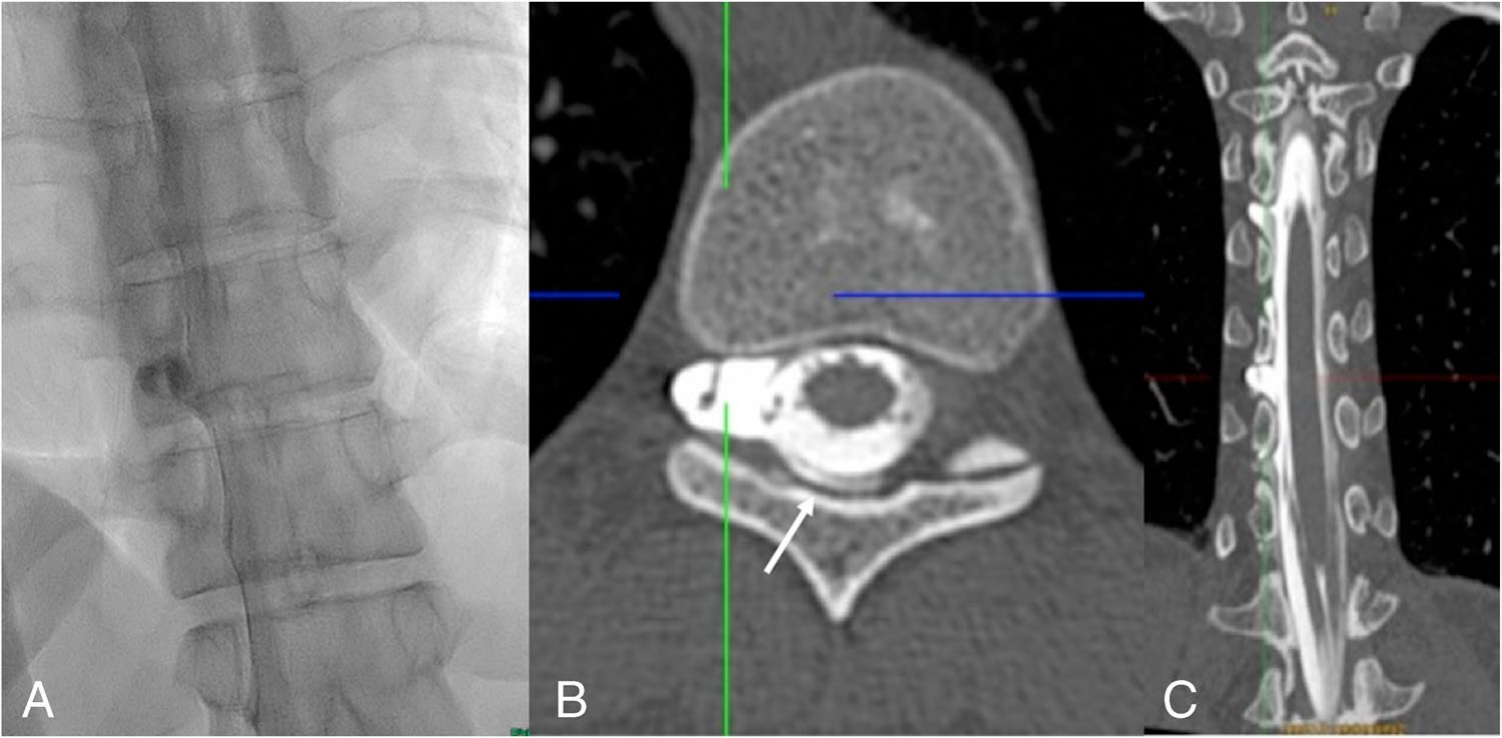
Type 2 leak: Right-sided lateral decubitus myelography shows a leaking meningeal diverticulum Th 10/11 on the right-side (A). Subsequent lateral decubitus CT myelography (B, C) shows extradural contrast (B: arrow) indicating a proximal leak
